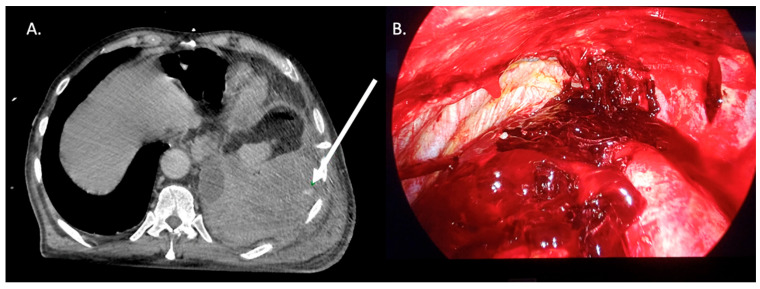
Left hemothorax due to intercostal artery bleeding in chest trauma. (A) CT scan showing the active bleeding (white arrow) and clots in the pleural space. (B) Intraoperative findings during VATS approach.
